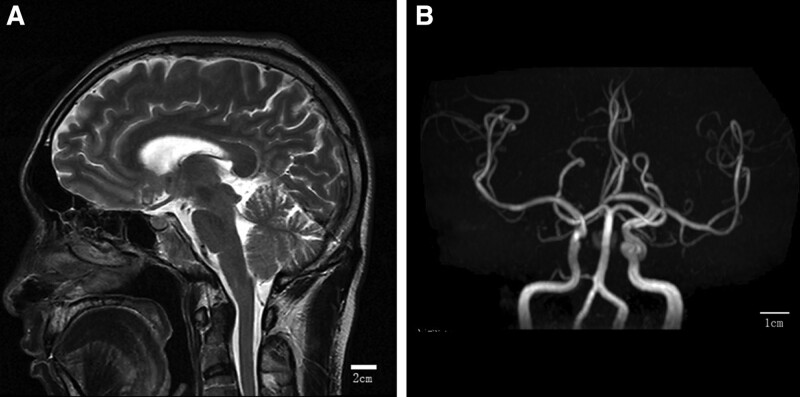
Imaging examination on admission showed no obvious abnormality in head MR. (A) MRI. (B) MRA. MRA = magnetic resonance angiography, MRI = magnetic resonance imaging.
